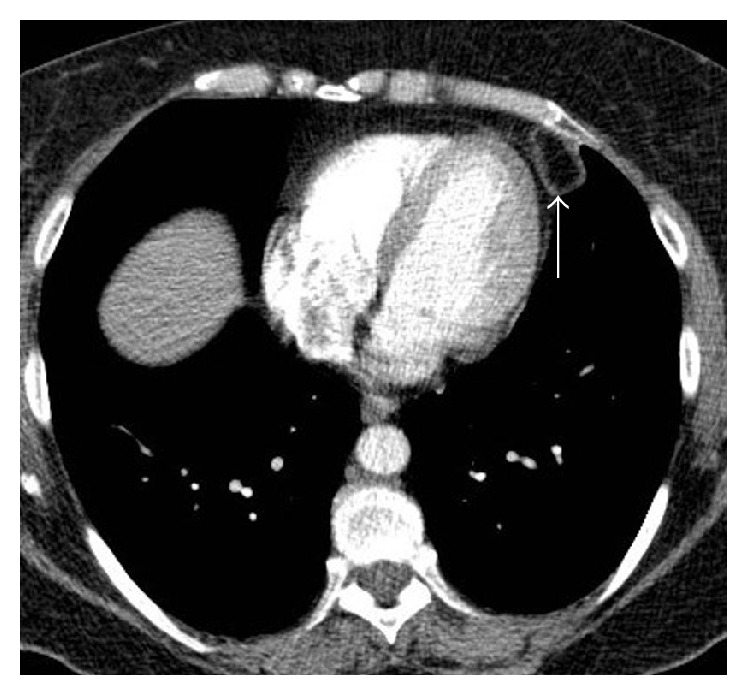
CT scan after 3 months showing a significant reduction in the size of the mass (arrow).
